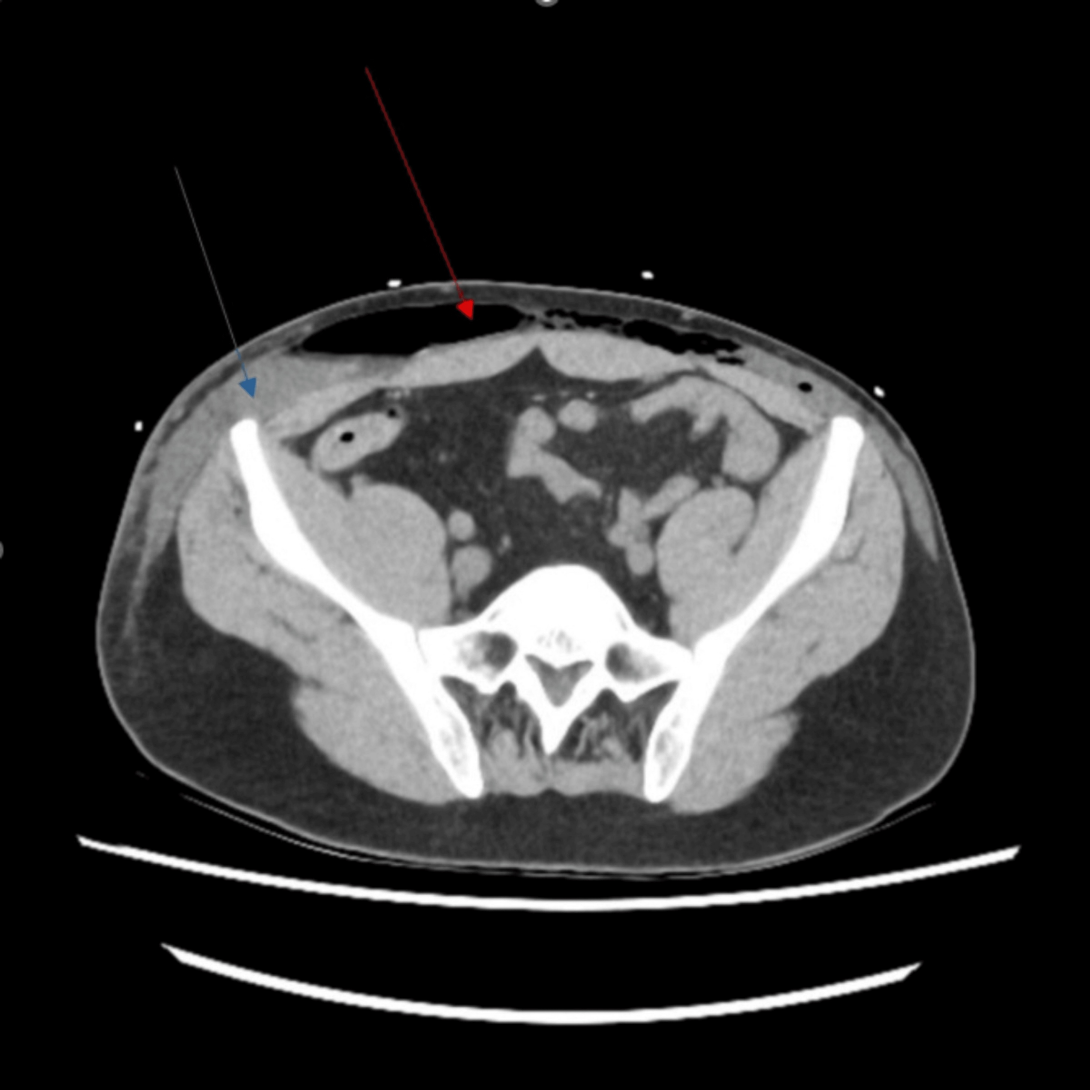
Axial view of CT abdomen demonstrating subcutaneous collection (highlighted by a blue arrow) and emphysema (highlighted by a red arrow) tracking down to the lower anterior abdominal wall and bilateral flanks, raising initial suspicion of necrotizing fasciitis.
